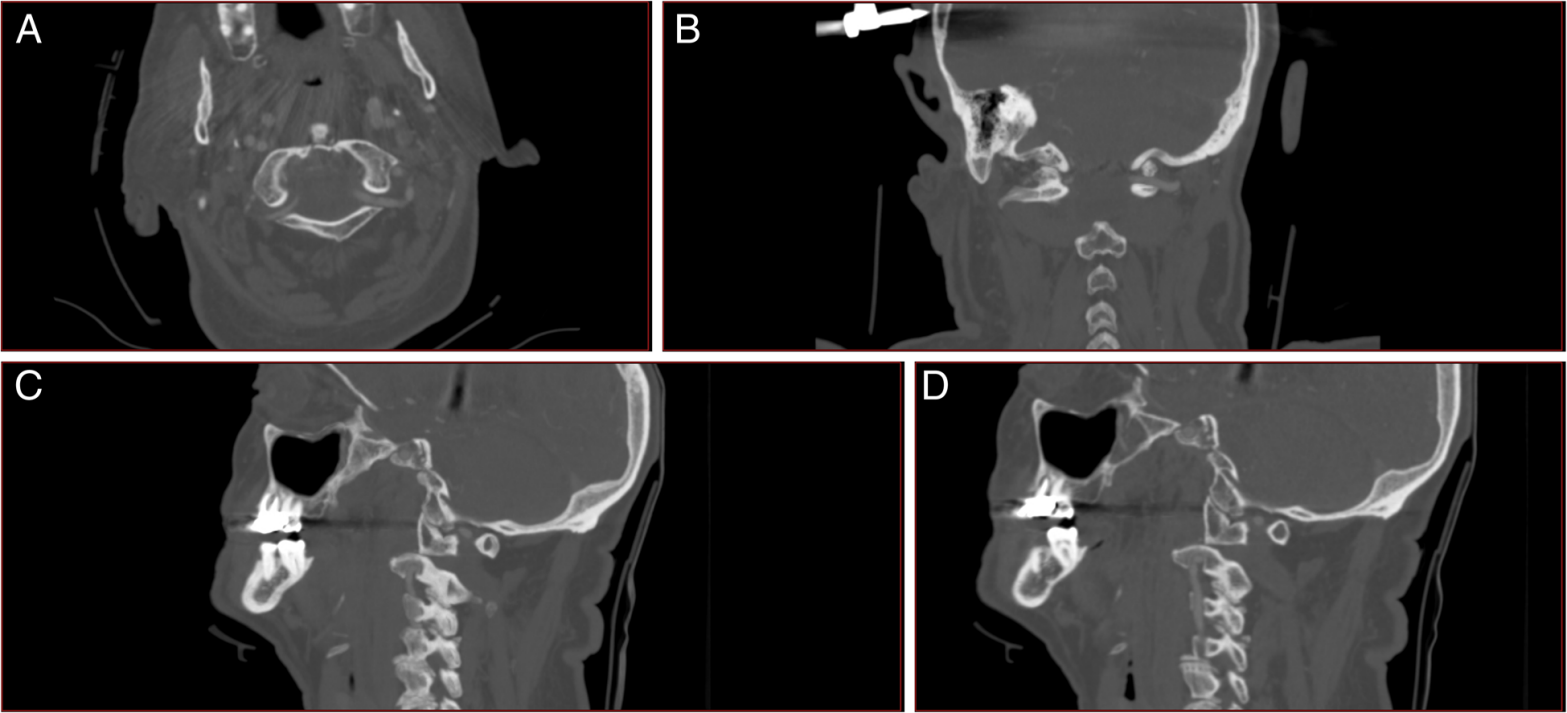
CT-angiography of the cervical spine showing maintained perfusion of the vertebral arteries. No signs of thrombosis or dissection. The left vertebral artery is dominant. a. Axial slice through the lateral masses of C1. b. Coronal slice through the 3rd segment of the vertebral arteries. c. Left-sided parasagittal slice through the dislocated C1-C2 joint. d). Right-sided parasagittal slice through the dislocated C1-C2 joint
